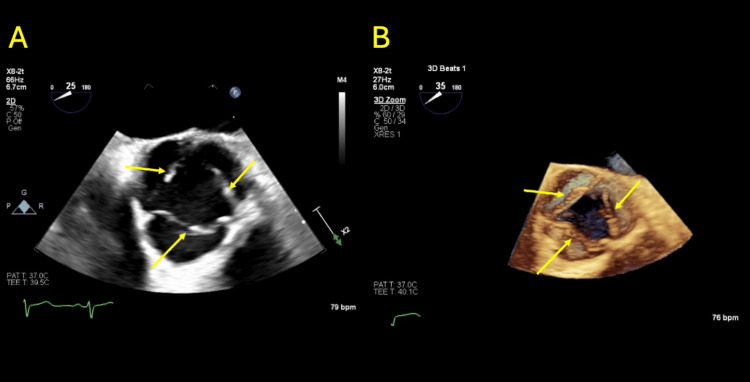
Mid-esophageal transesophageal echocardiogram short-axis view of the aortic valve On both 2D echocardiographic view (A) and 3D echocardiographic view (B), there are multiple small, nodular, sessile excrescences along the lines of coaptation, likely nonbacterial thrombotic (marantic) vegetations (denoted by yellow arrows).
